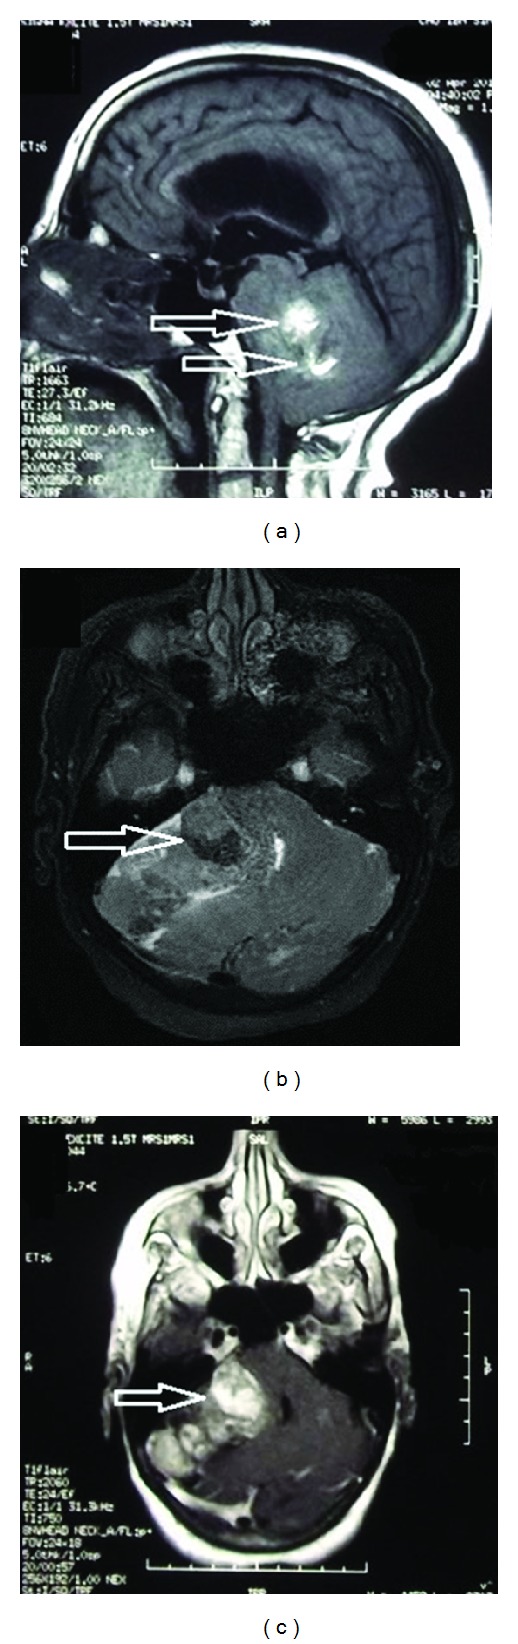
MRI of the brain in sagittal T1 (a), axial fat sat sequence (b), and axial T1 with gadolinium, respectively (c). Note the fat component (arrows in (a) and (c)) that turns hypointense after fat exclusion (arrow in (b)).
